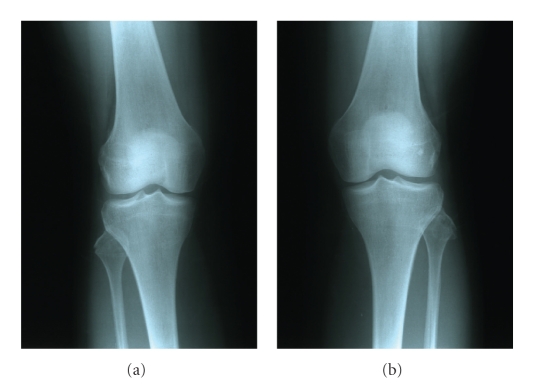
Anteroposterior radiographs of both knees 6 months after trauma. Fractures of both fibular heads are fully united.
